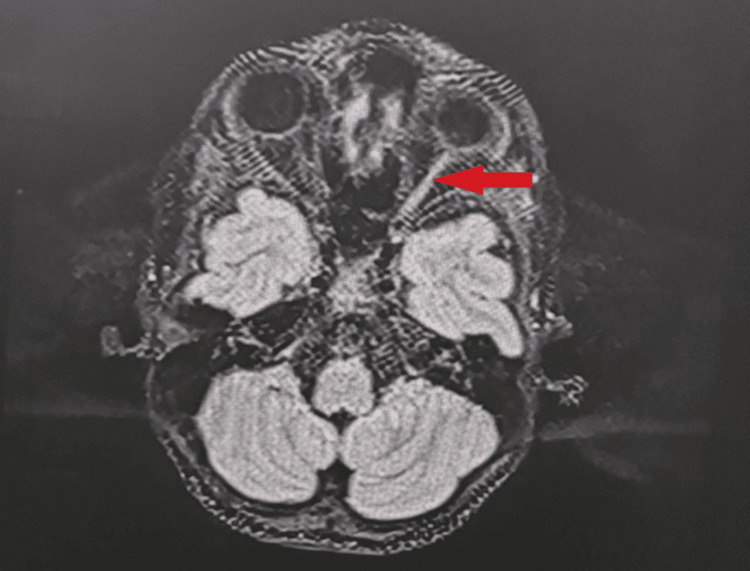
Axial T2-weighted brain MRI demonstrating left optic nerve involvement. The left optic nerve shows a hyperintense signal (red arrow) consistent with optic neuritis. The asymmetric optic nerve hyperintensity corresponds to the patient’s complete left eye visual loss and subsequent optic atrophy. MRI: magnetic resonance imaging
